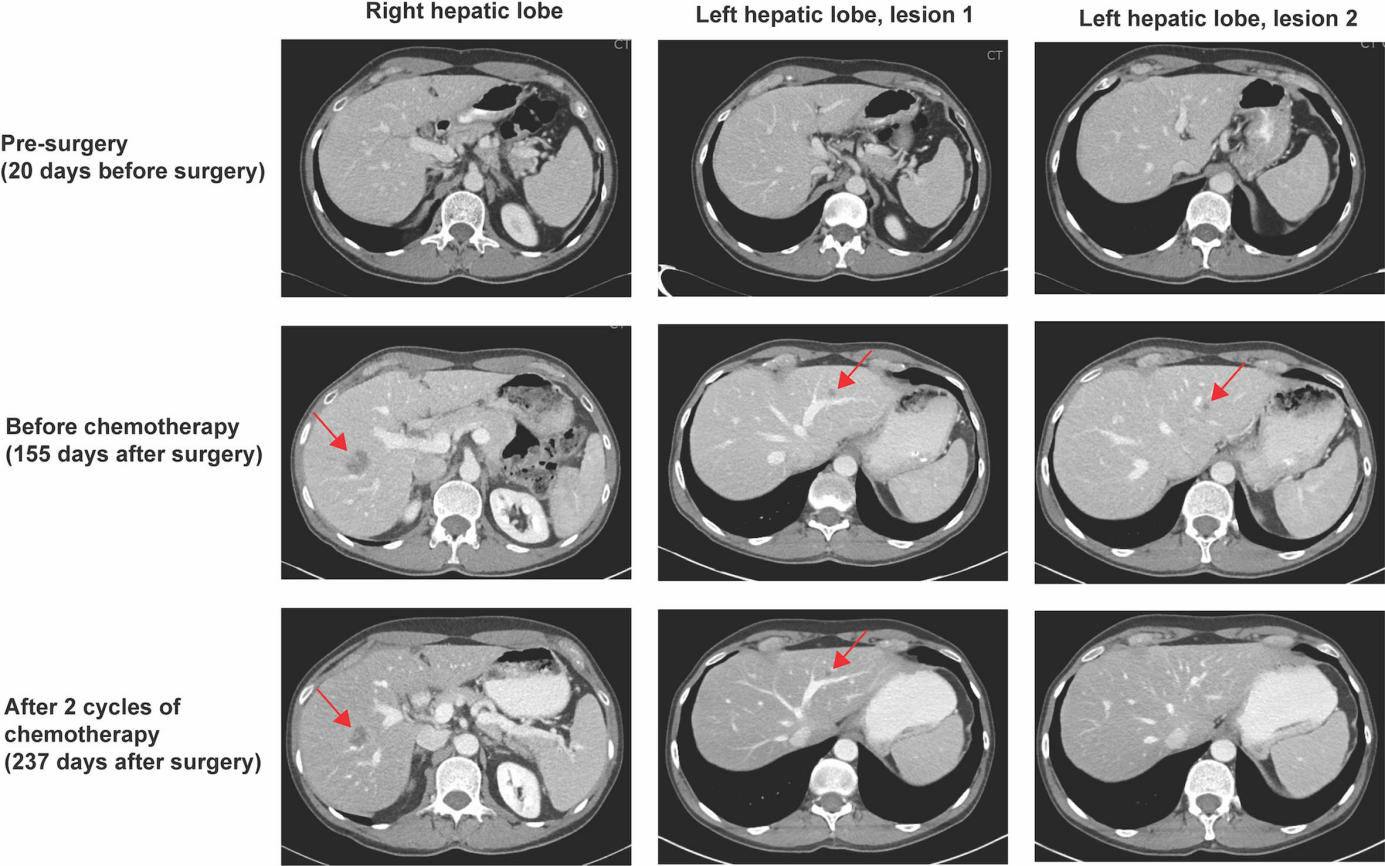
CT scan results. CT scan 20 days prior to right hemicolectomy did not show any evidence of metastatic disease (top panel). Rising ctDNA levels prompted a CT scan on post-operative day 155, which revealed a 2.4 x 1.8 cm ill-defined hypoattenuating lesion in the right hepatic lobe, as well as 0.8 cm and 0.4 cm nodules in the left hepatic lobe (red arrows, middle panel). An interval CT scan after two cycles of chemotherapy showed response of all hepatic lesions (red arrows, bottom panel).
